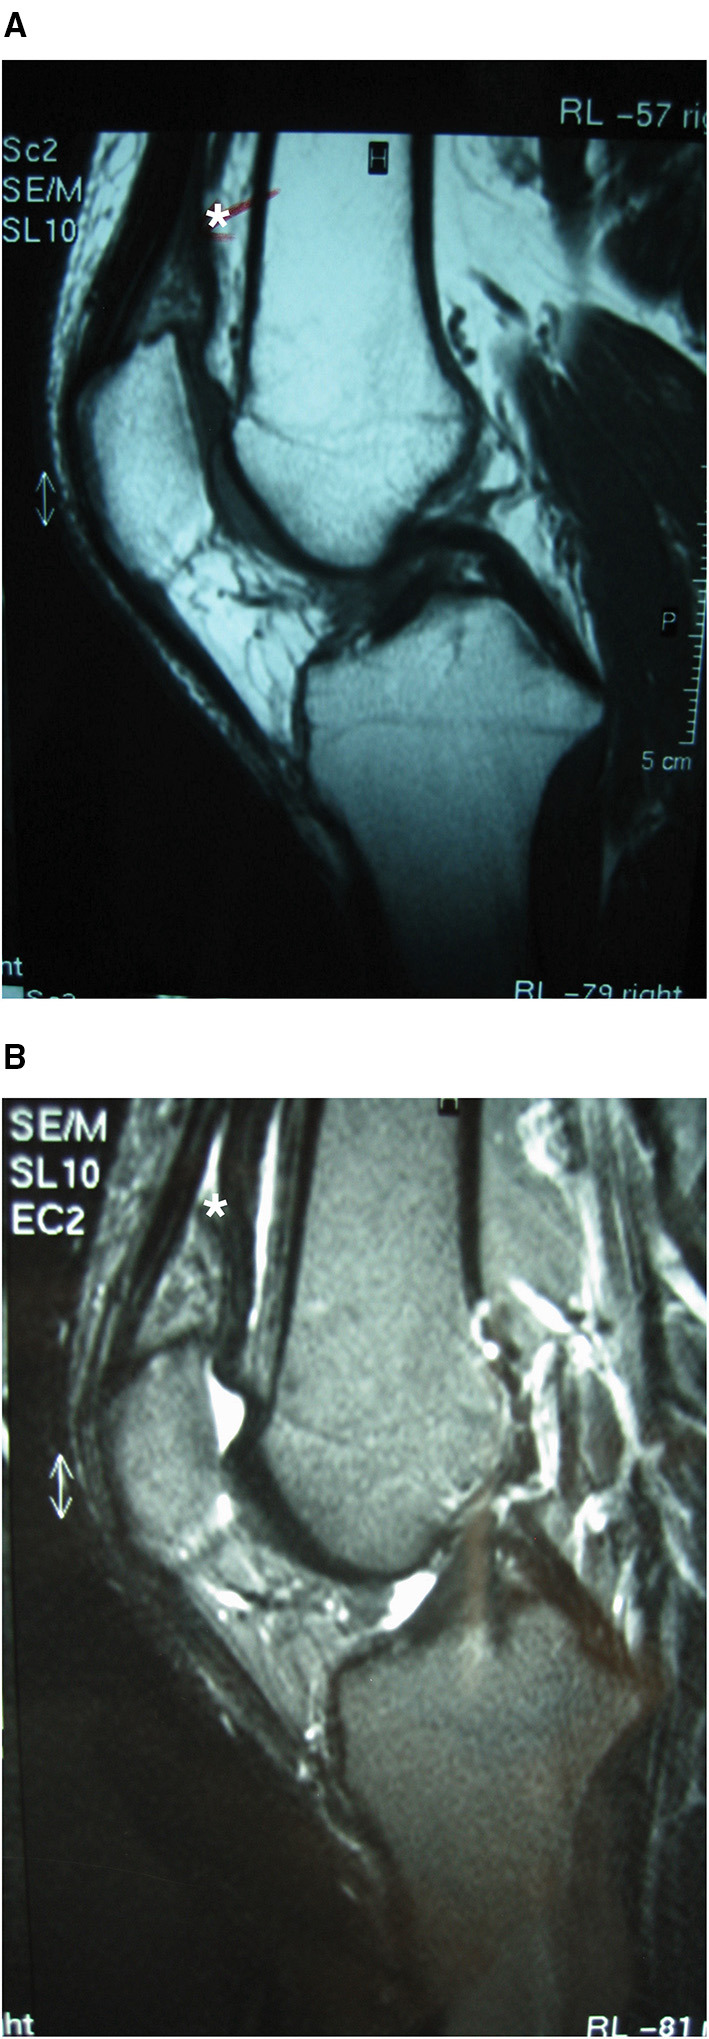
Case 3: suprapatellar SH MRI. MR sagittal images showing an intra-articular synovial mass located superior and medial to the patella that eroded the superior patellar cortical bone; the mass was heterogeneously hypointense on T1-weighted imaging (A) and isointense on T2-weighted imaging (B). *Location of the Synovial Haemangioma tumor in the knee.
